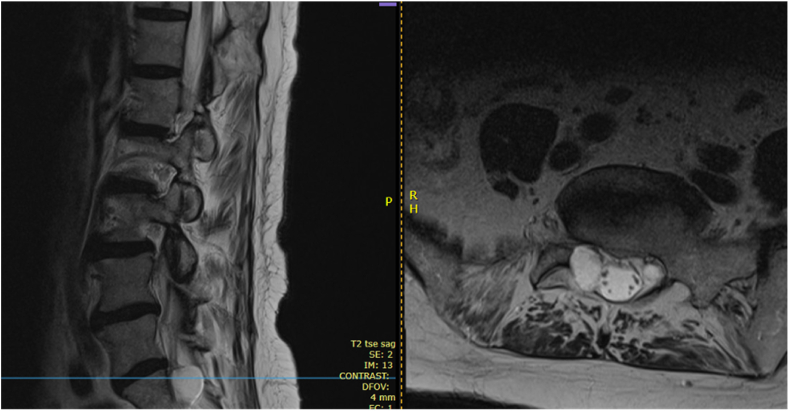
Sagittal and axial views of lumbosacral MRI demonstrating the superior portion of a Tarlov cyst at S1.
